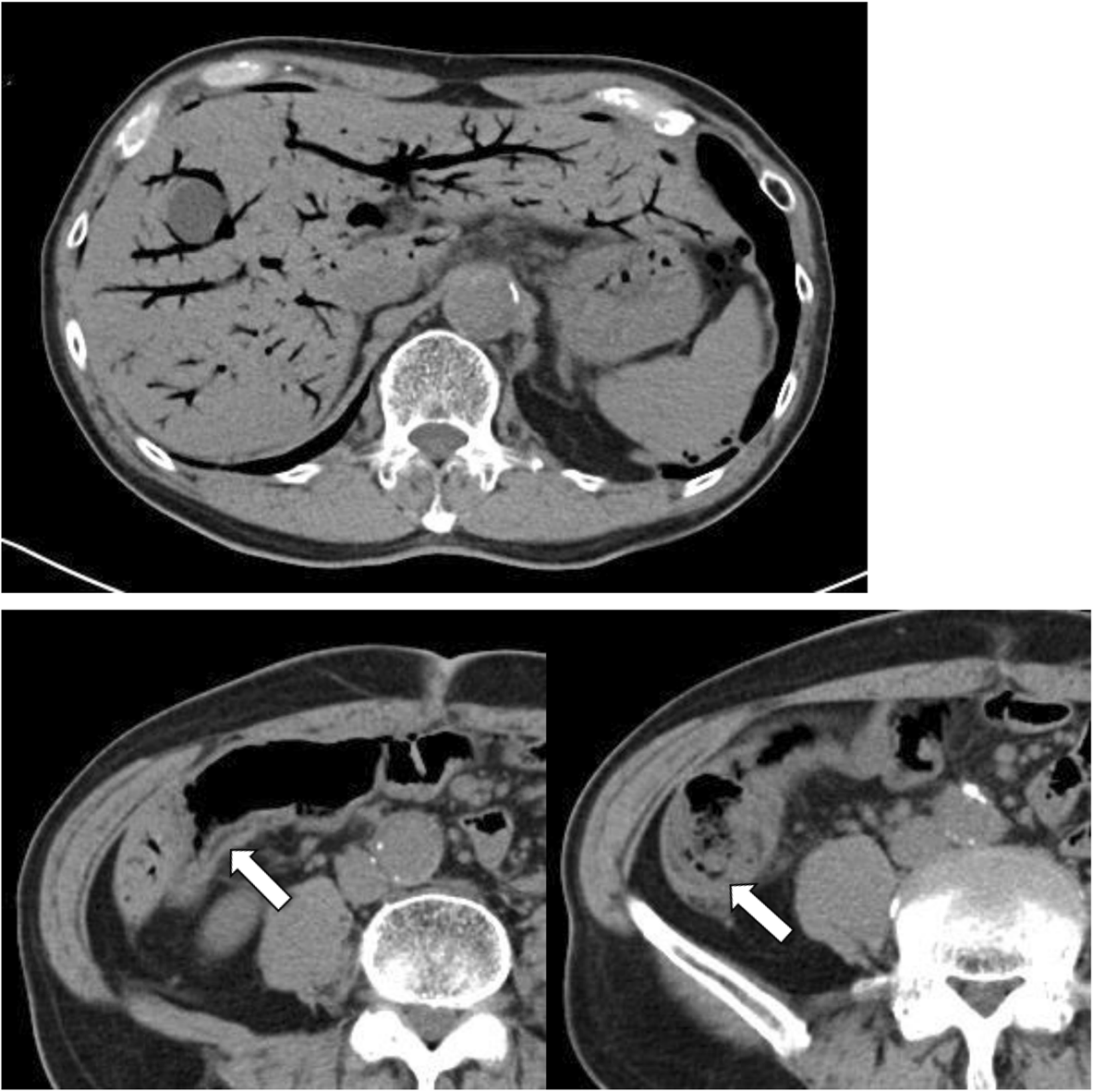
CT 6 days after the operation. CT showed portal venous gas over the entire area of the liver. The arrow shows pneumatosis intestinalis in the thick wall of the ascending colon
